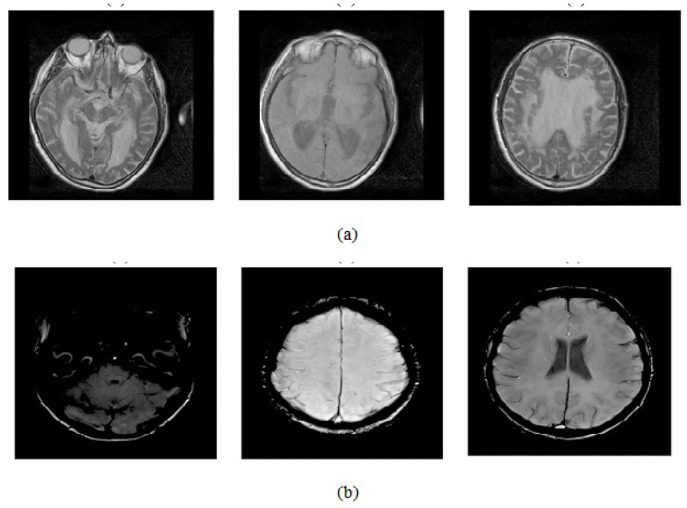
MRI images (a) PD patients, (b) normal people.
